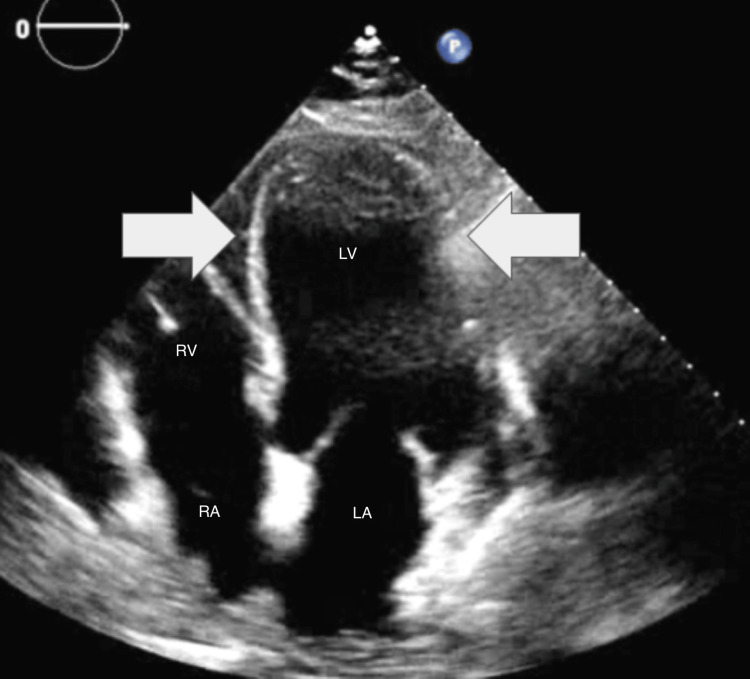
Apical four-chamber transthoracic echocardiogram (end-diastole frame) demonstrating apical ballooning and hypokinesis/akinesis LV: left ventricle, LA: left atrium, RA: right atrium, RV: right ventricle
